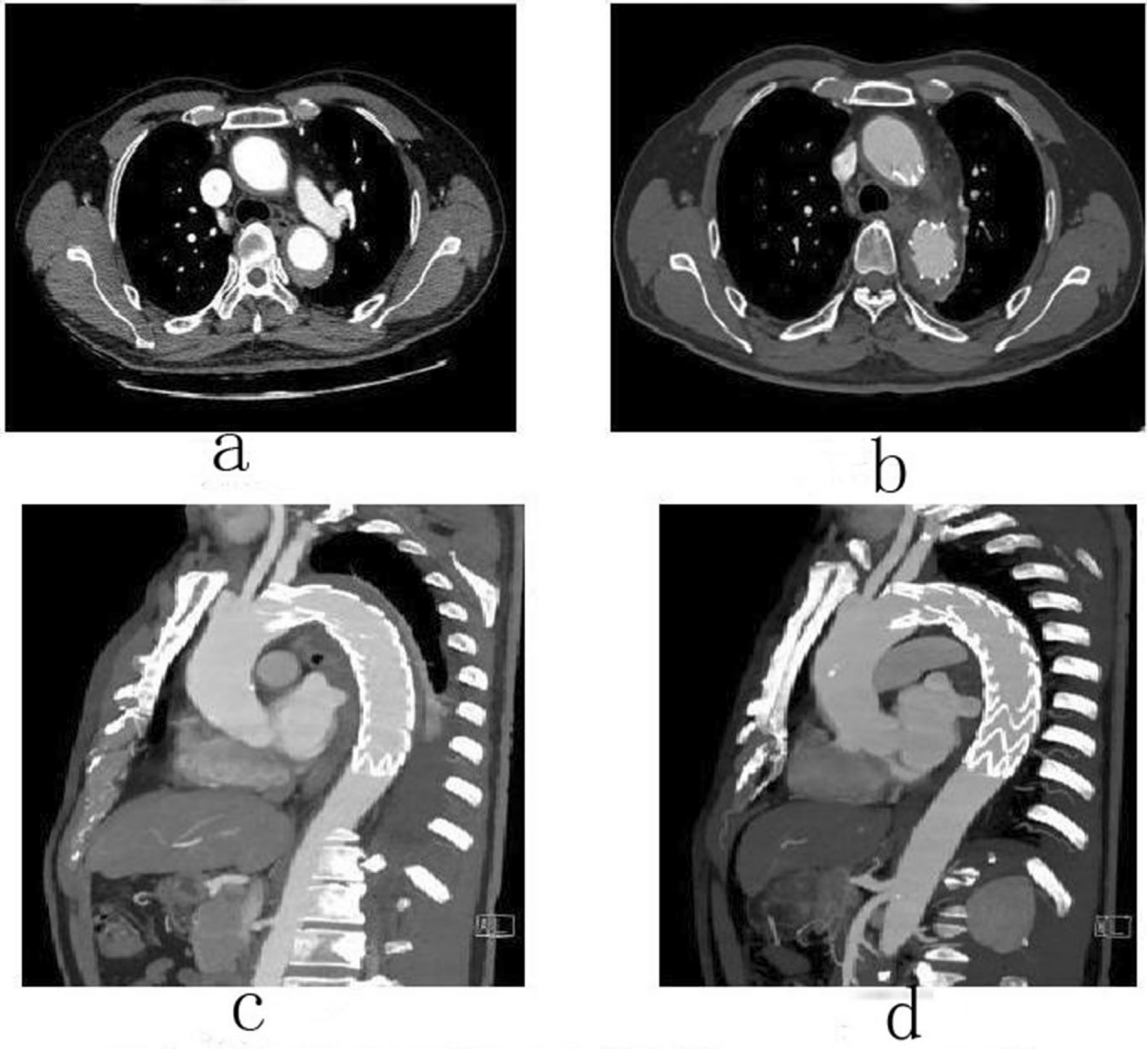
Male, 62 years old, admitted to hospital for “repetition of chest and back pain for 9 h”. Diagnosis: Standford A IMH TEVAR. CTA of the patient in different periods: (a) Admission; (b) 5 days after surgery; (c) 5 days after surgery; (d) 3 months after surgery.
